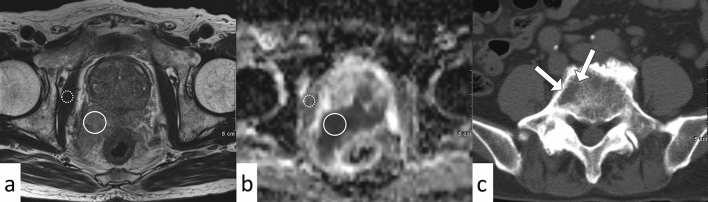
An 80-year-old man with prostate cancer with an osteolytic bone metastasis (prostate-specific antigen density = 5.6). Prostate MRI shows the primary tumor (Gleason score 9), with normalized T2 signal intensity of 3.2 (a) and normalized apparent diffusion coefficient of 0.50 (b). Axial CT reveals an osteolytic lesion that is indicative of bone metastasis (c, arrow).
